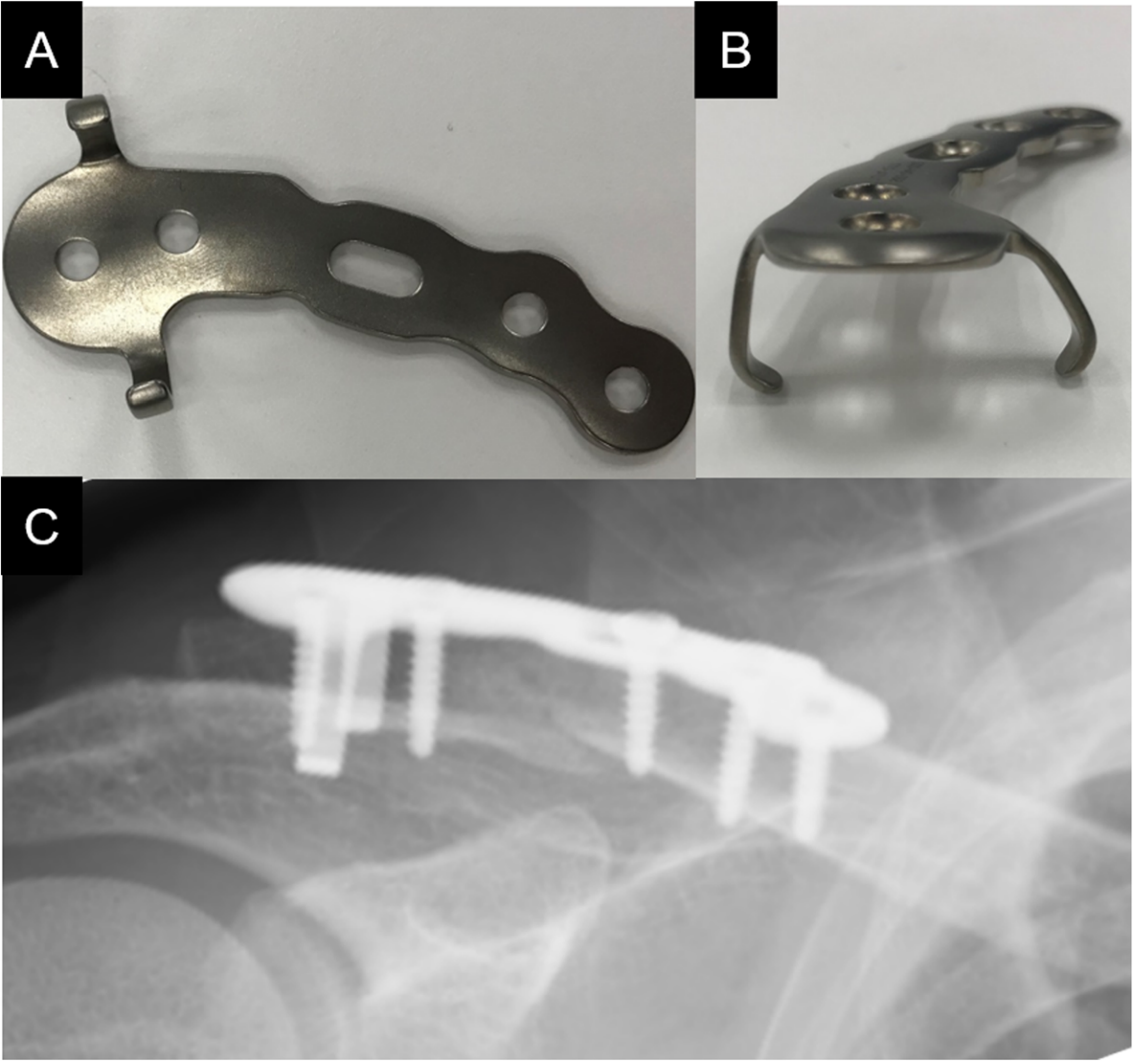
The photo images demonstrate a SCORPION® plate (a and b). Plain radiograph showing osteosynthesis using a SCORPION® plate within six days after injury (c)
